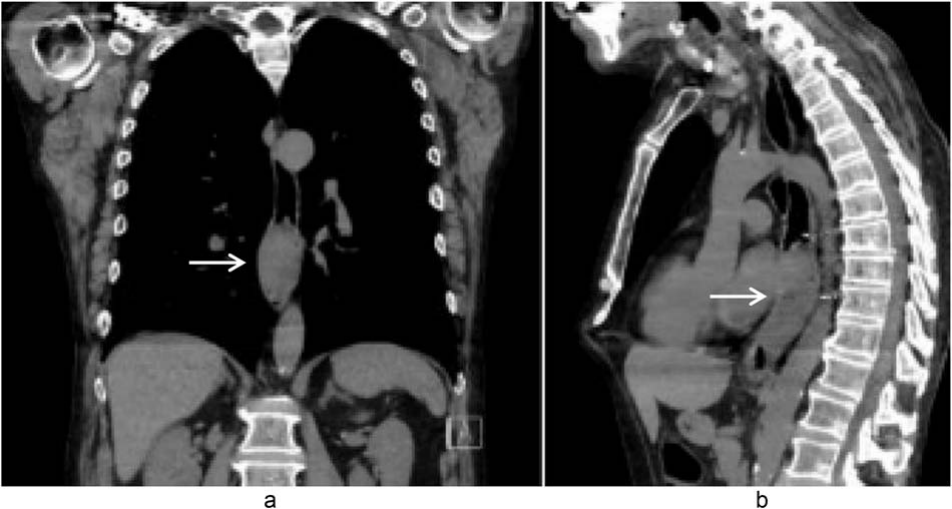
Coronal (a) and sagittal (b) views of the initial CT scan at time of presentation that revealed a large esophageal mass with concern for complete obstruction (arrows).
